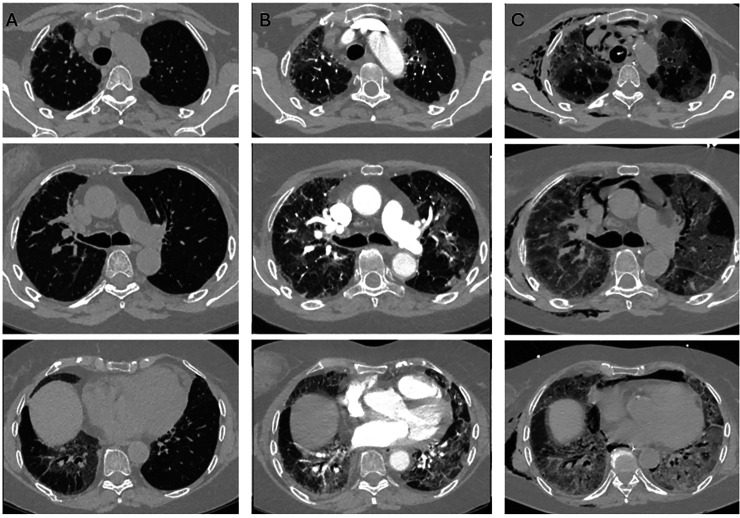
Computed tomography images from patient 5. (a) Baseline scan before COVID-19, performed for follow-up of pre-existing fibrosis. The patient had previously undergone unilateral lung transplantation due to fibrotic NSIP. (b) Day 6 after clinical onset: bilateral GGOs, early reticulations, and focal areas of OP. (c) Day 18 after clinical onset (day of death): marked progression of GGOs. Reticulations and bronchial dilatation had increased in both lungs, with widespread crazy-paving pattern. Notably, no discernible difference in pattern or extent was observed between the transplanted and native lungs, and the caliber pre-existing traction bronchiectasis in the native lung remained unchanged. GGO, ground-glass opacity; NSIP, non-specific interstitial pneumonia; OP, organizing pneumonia.
